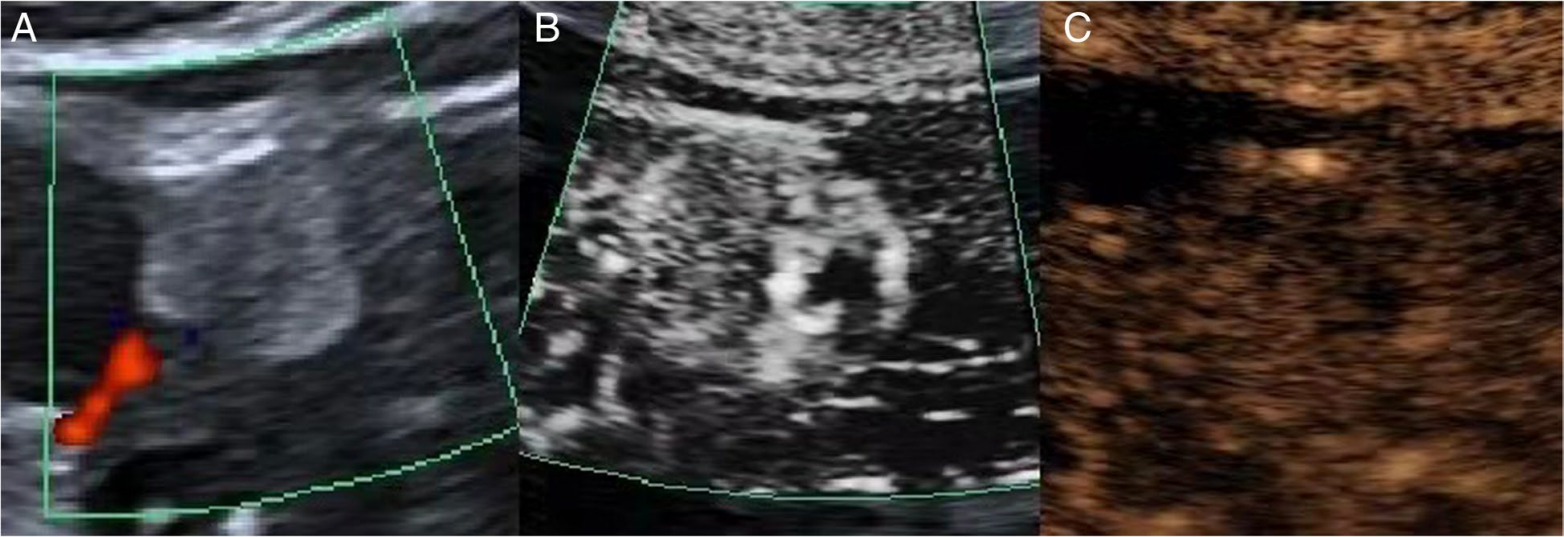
A solid mass of left kidney A ultrasound imaging of CDFI, hyperechoic mass with clear boundary, regular shape, and inhomogeneous internal echo B SMI show point-line blood flow signal in the tumor, blood flow grade 1. C MFI show point-line blood flow signal in the tumor, blood flow grade 1, pathological result was hamartoma
